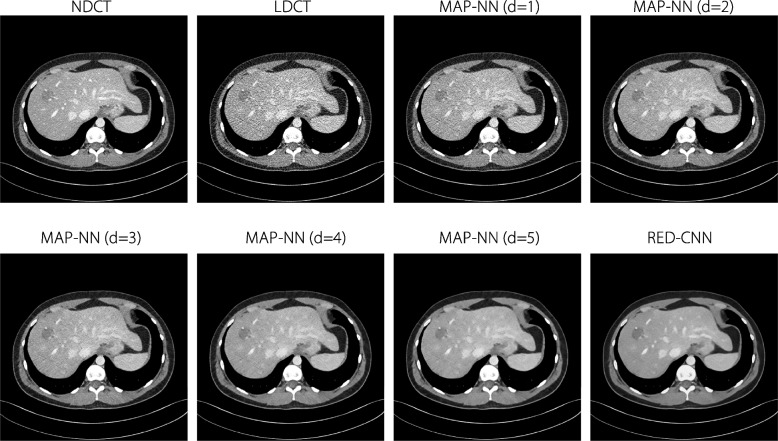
Examples of images from the CT-IQA dataset
